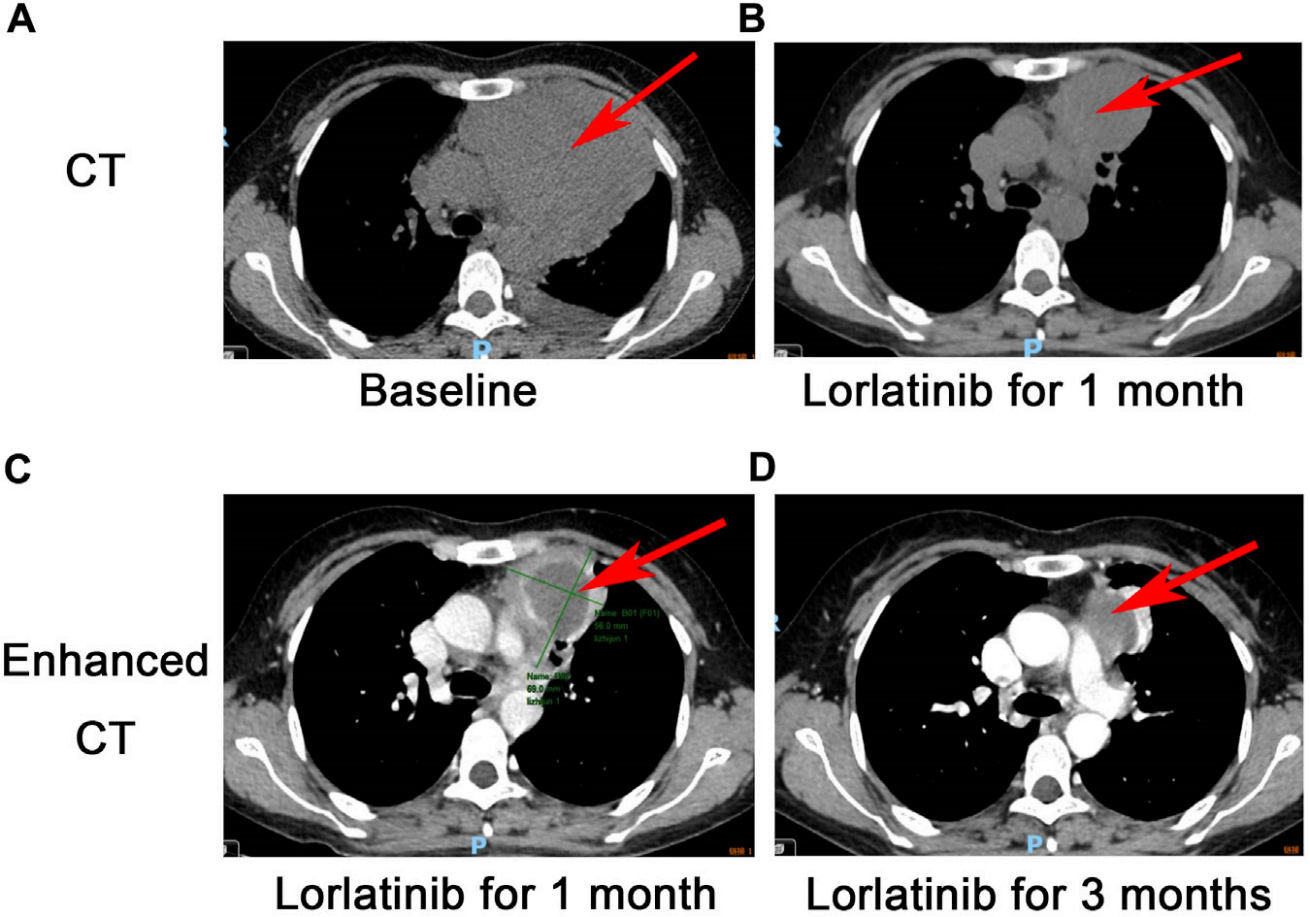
CT scan for EIMS: A large mass with unclear boundaries in the left anterior mediastinum measures 10.1 * 9.4 cm (A), a partial response was revealed after 1 month following lorlatinib (B), Comparison of contrast-enhanced CT of the chest at 1 month (C) and 3 months (D) after treatment with Lorlatinib.
